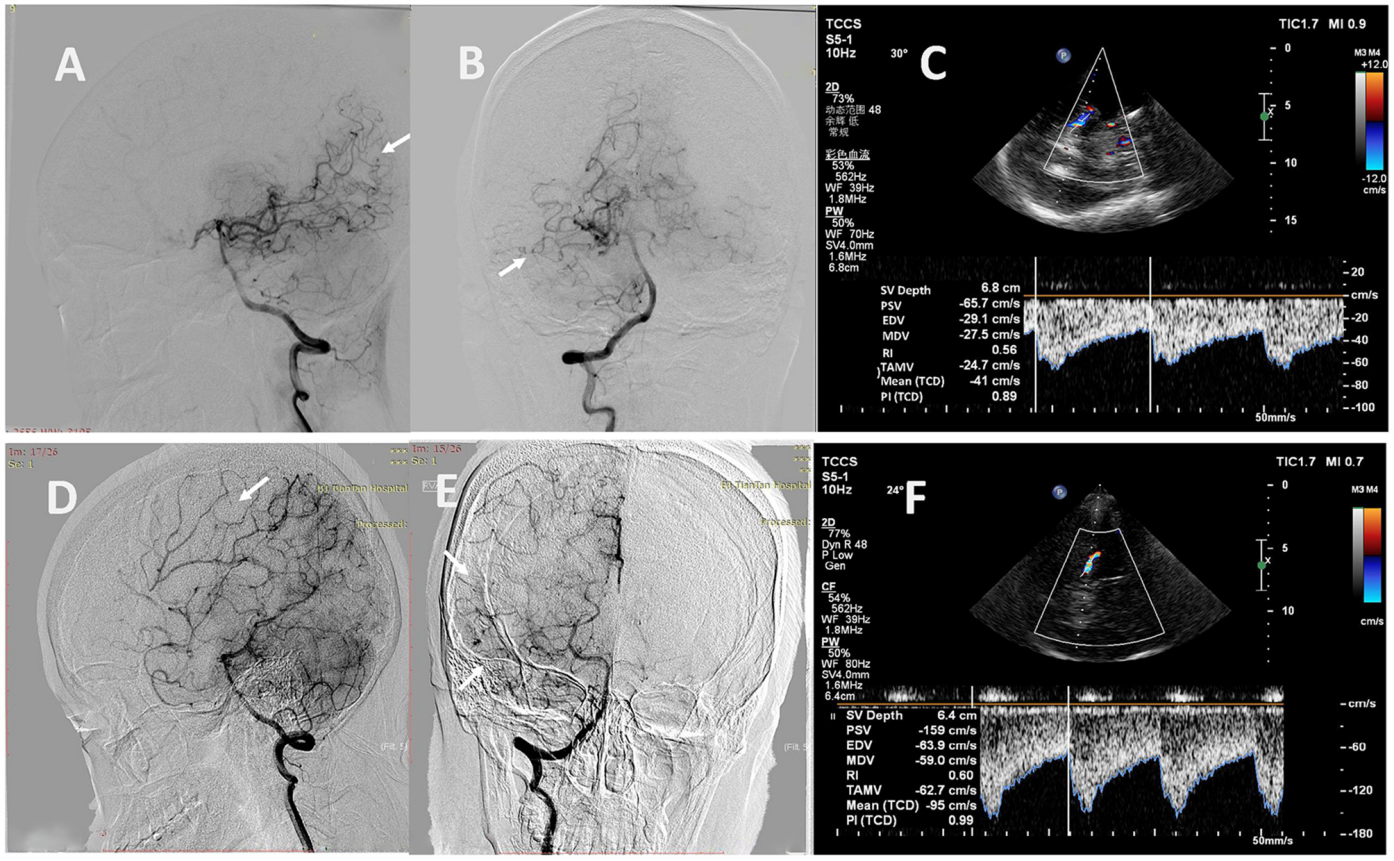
Representative cases. (A, B) A 24-year-old man with MMD scored 2 points in the leptomeningeal system on the right (white arrows). (C) The TCCS showed low values of PSV (66 cm/s) and EDV (29 cm/s) in the right PCA. (D, E) A 33-year-old woman with MMD scored 5 points in the leptomeningeal system on the right (white arrows). (F) The TCCS showed high values of PSV (159 cm/s) and EDV (64 cm/s) in the right PCA. MMD, moyamoya disease; TCCS, transcranial color-coded duplex sonography; PSV, peak systolic velocity; EDV, end diastolic velocity; PCA, posterior cerebral artery.
